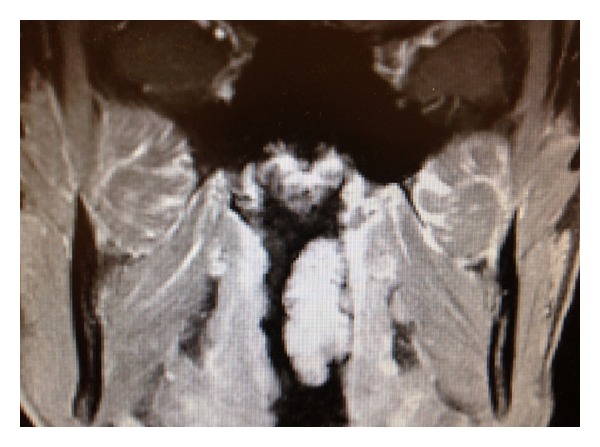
MRI coronal image demonstrating a pedunculated 3.1 × 1.7 × 1.2 cm nonvascular mass emanating from the left nasopharynx.
